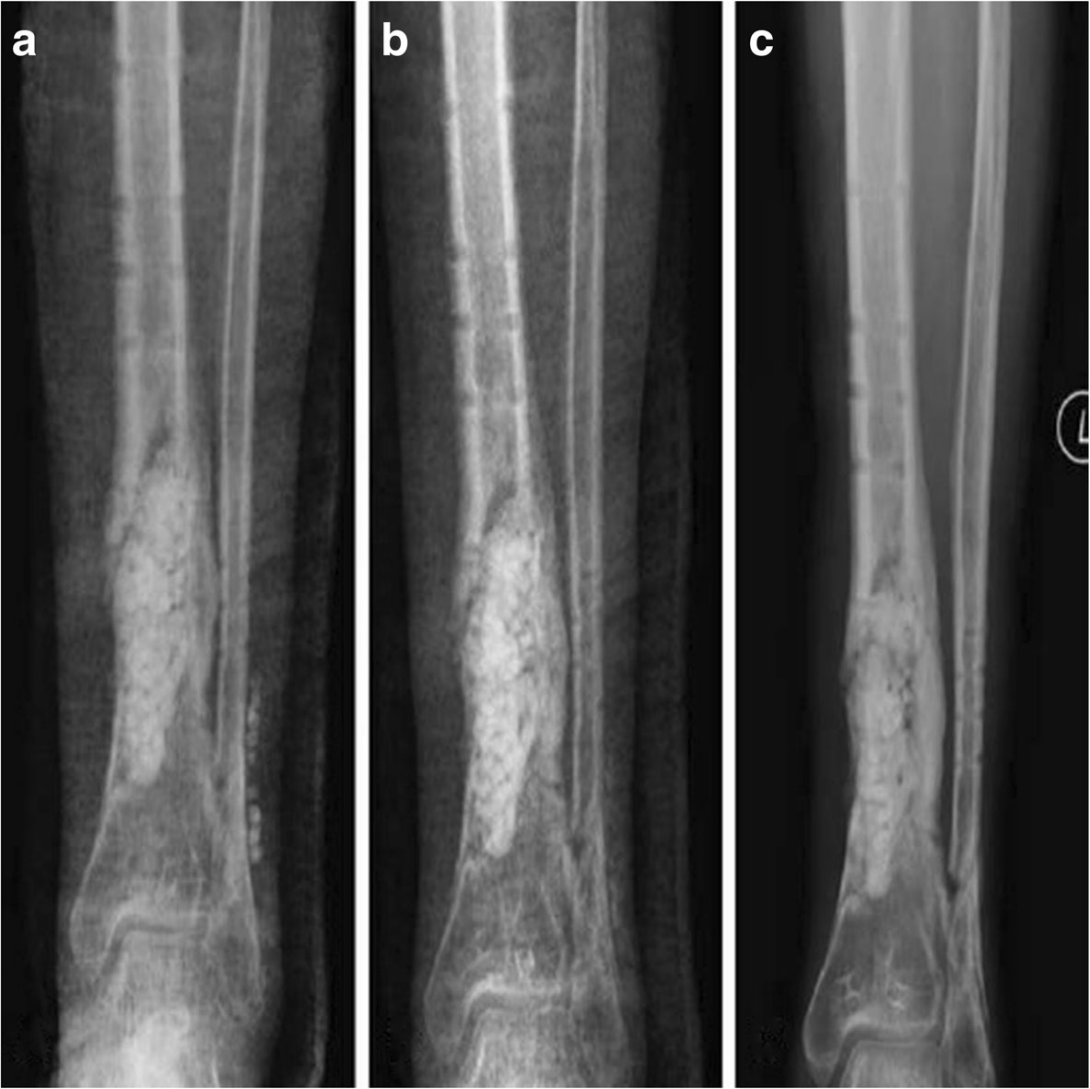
a-c Postoperative radiographs showed that the combination of calcium sulfate pellets and PMMA spacer was filled in the bone cavity after surgical treatment, and the calcium sulfate pellets were absorbed at an average of 6 weeks (range, 30–60 days)
